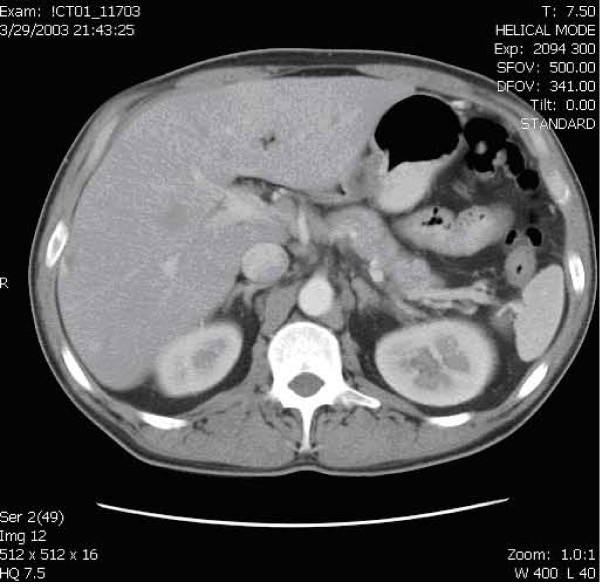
CT of the abdomen of the same patient 18 months earlier. No pancreatic fluid collection is seen.
